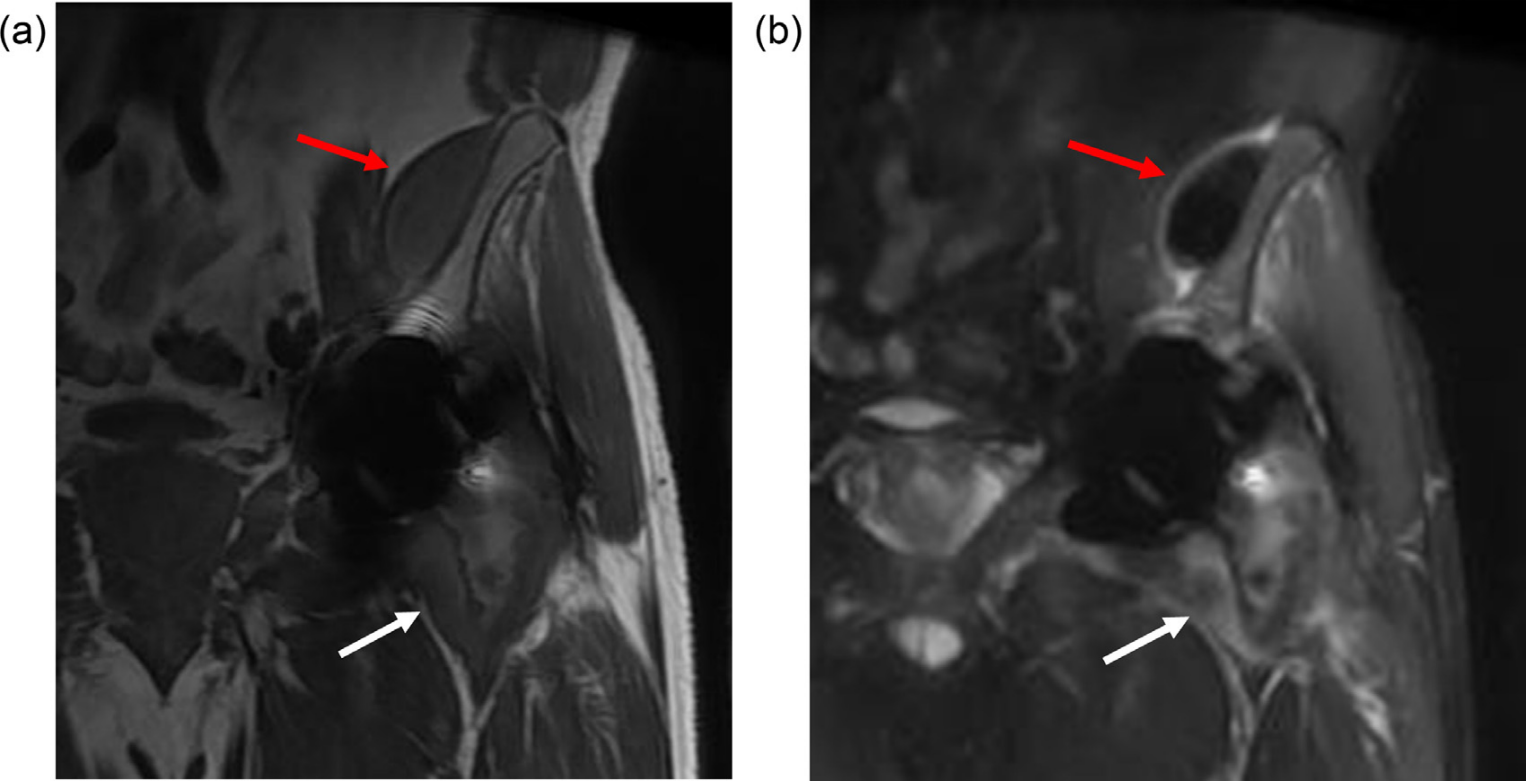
(a) Coronal T1 and (b) coronal STIR images from MRI of the left hip. These demonstrate a low signal intensity structure along the medial aspect of the left iliac bone, compatible with metallic debris (red arrows). The left hip arthroplasty is seen with distention of the joint capsule, particularly along the medial aspect of the femoral neck (white arrows).
